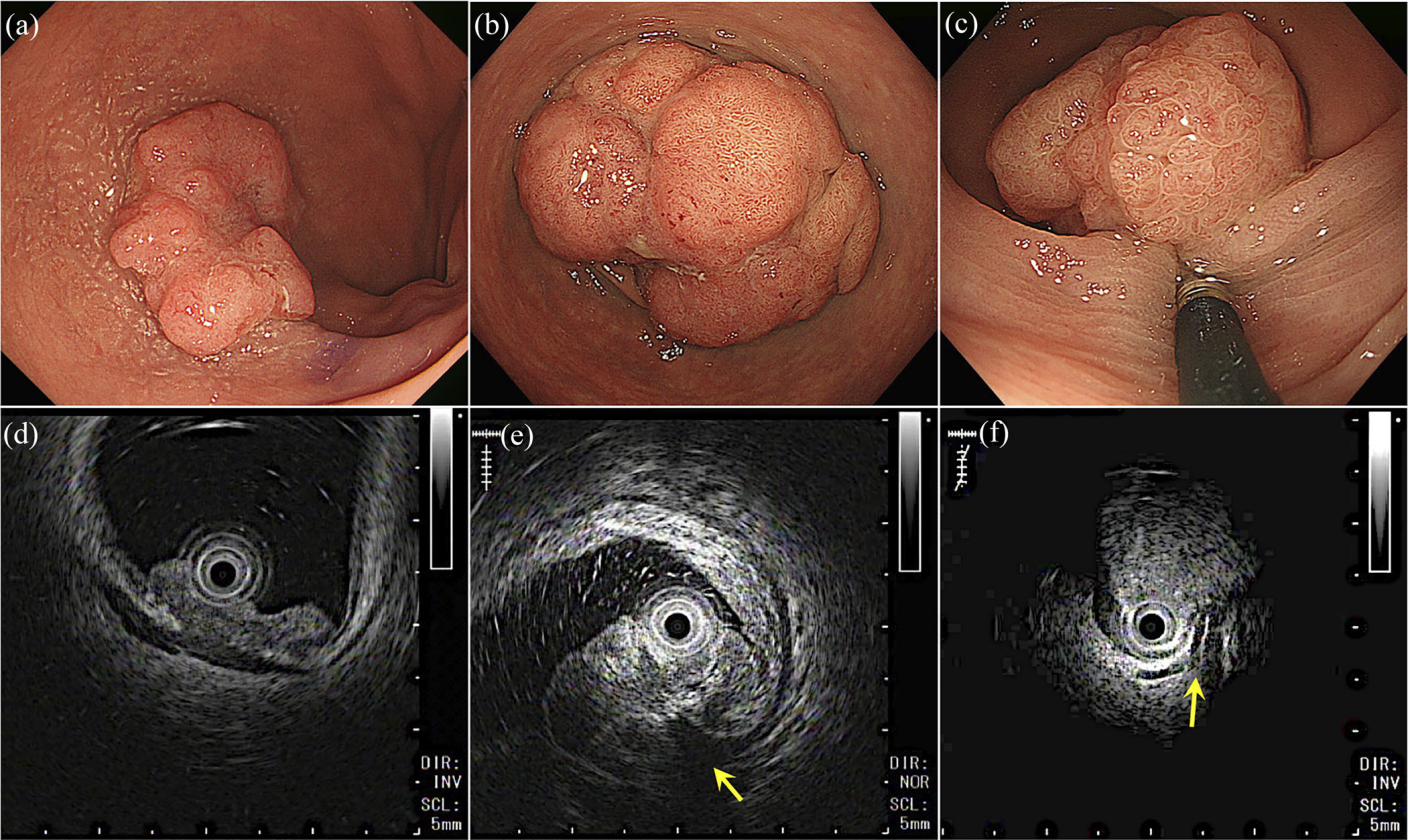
Images of the endoscopic ultrasonography muscle‐retracting (EUS‐MR) sign. Endoscopic images (a–c) and corresponding endoscopic ultrasonography images (d–f). Although the tumor is invading the low echoic layer corresponding to the muscle layer, the EUS‐MR sign is negative because the muscle layer is stretched (d). The EUS‐MR sign is positive when the low echoic layer corresponding to the muscle layer is pulled toward the mucosal lesion (yellow arrow e, f).
